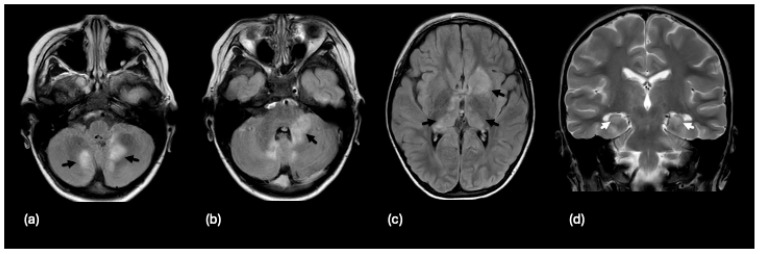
A 4-year-old male with recent viral encephalitis presenting with new gait ataxia, finger tingling, and headache and positive MOG antibody. Axial FLAIR images demonstrate multifocal, non-enhancing lesions (black arrows) in the (a) cerebellum, (b) left brachium pontis, and (c) deep gray matter structures, including the left caudate, anterior left putamen and globus pallidus, and the right greater than left thalami. Additional bilateral hippocampal swelling and signal abnormalities (white arrows) are shown on (d) coronal T2-weighted images.
